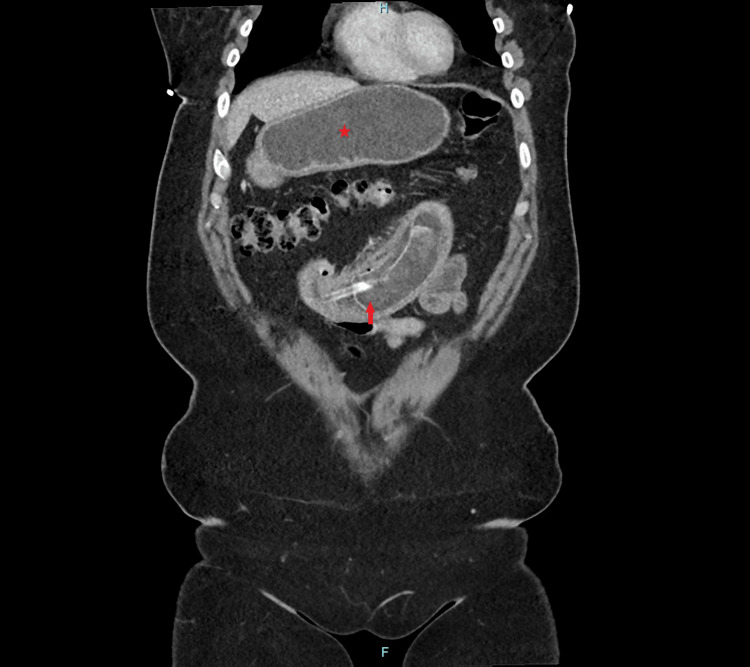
Coronal computed tomography image showing marked dilatation of the stomach, along with a device consisting of a catheter and a fluid-filled balloon in the proximal jejunum (arrow) The star indicates the distended stomach. The arrow points to the fluid-filled balloon in the proximal jejunum.
